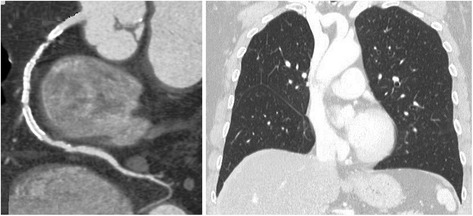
Representative case of follow-up evaluation of a patient with previous percutaneous coronary intervention and previous lung lobectomy. The cardiac CT revealed a significant in-stent restenosis in the proximal segment of the right coronary artery (left upper panel). Thoracic evaluation showed no recurrence 5 years after right upper lobectomy (right panel)
